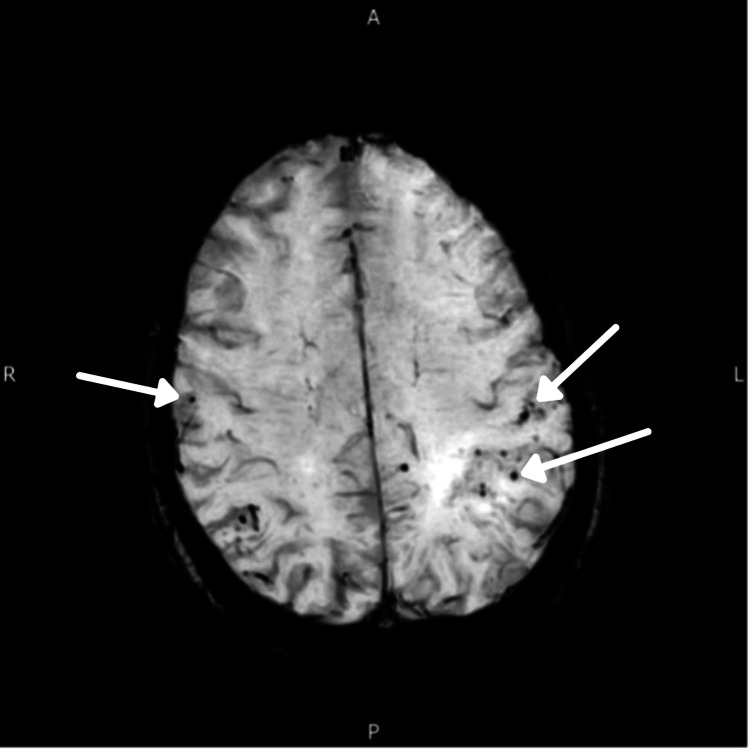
MRI June 2020 was consistent with a CAA pattern (microbleeds; arrows). CAA, cerebral amyloid angiopathy; MRI, magnetic resonance imaging
